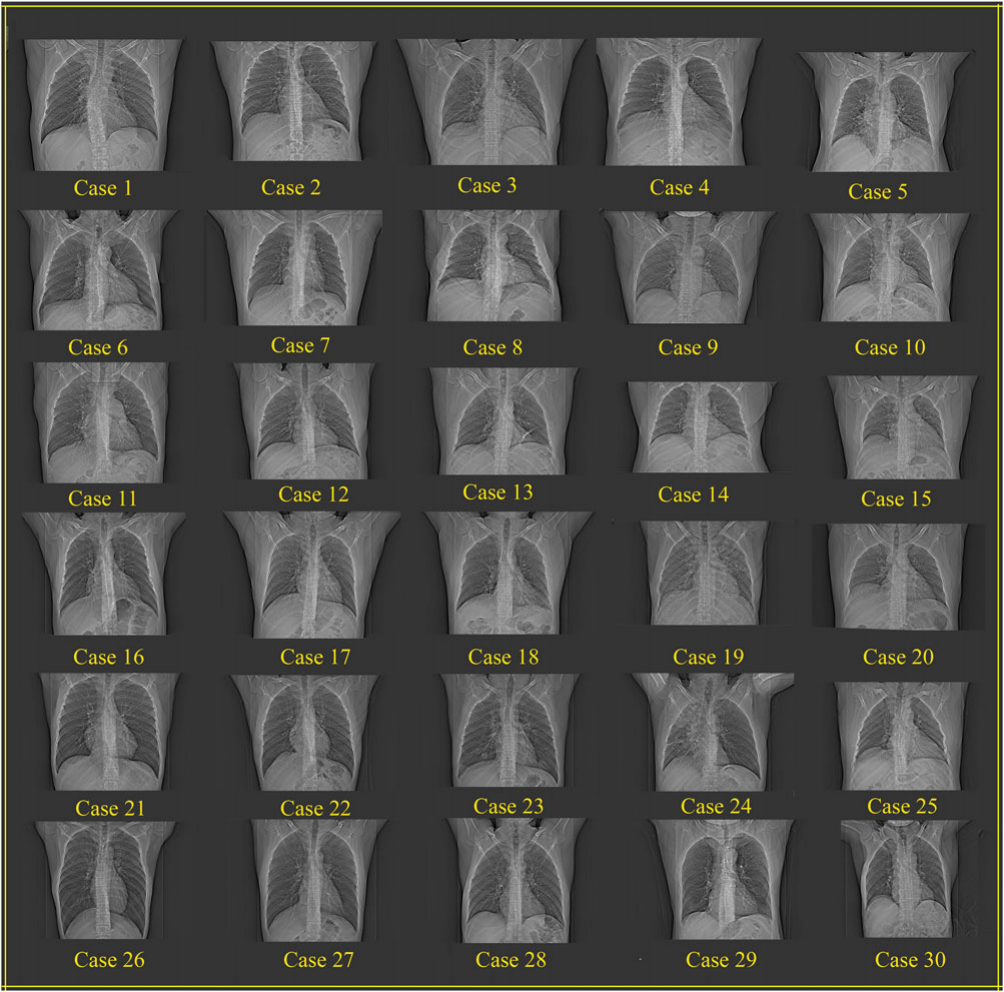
Comparison of the lung size of 30 normal patients in radiography images. The cases that have been placed in a particular row belong to the same height/weight percentile. The variation in lung volume for the patients in any given row is clear.
